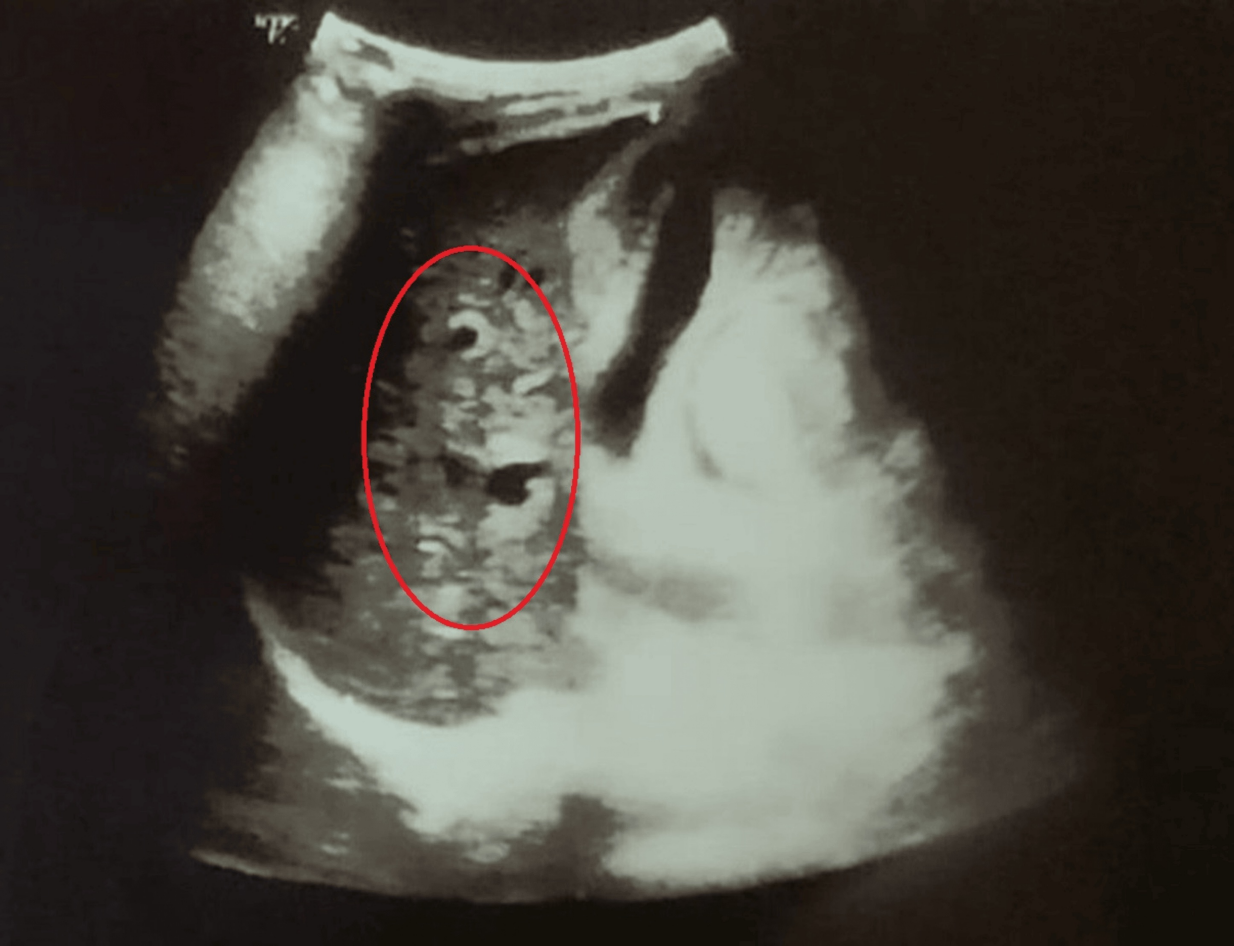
Abdominal ultrasound showing increased liver echotexture (red oval)
